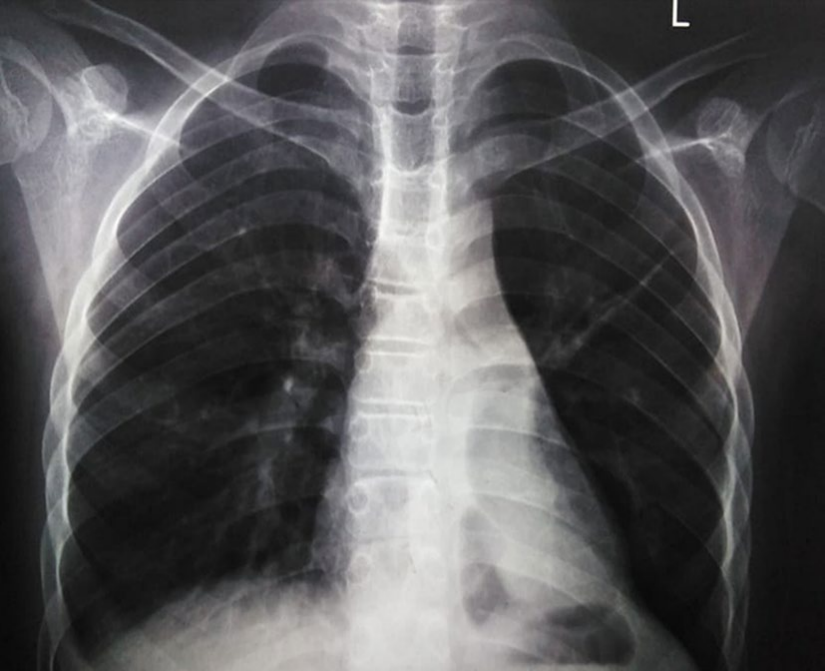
Chest X-ray showing a normal left lung at 24 h. after a flexible bronchoscopy
